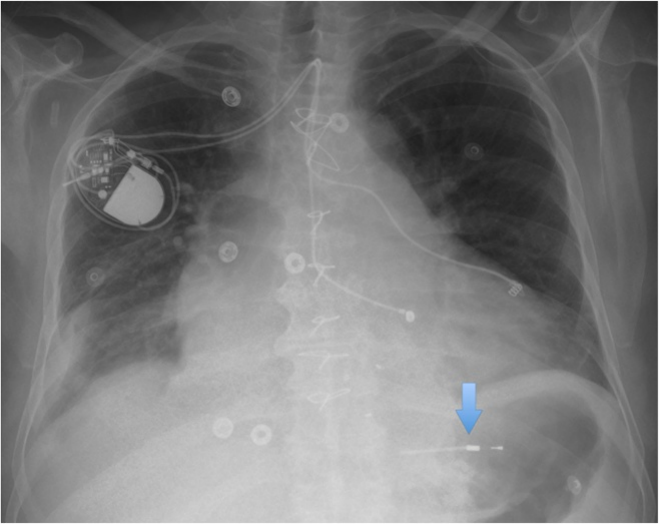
Postoperative chest X-ray. Blue arrow: residual lead fragment.
